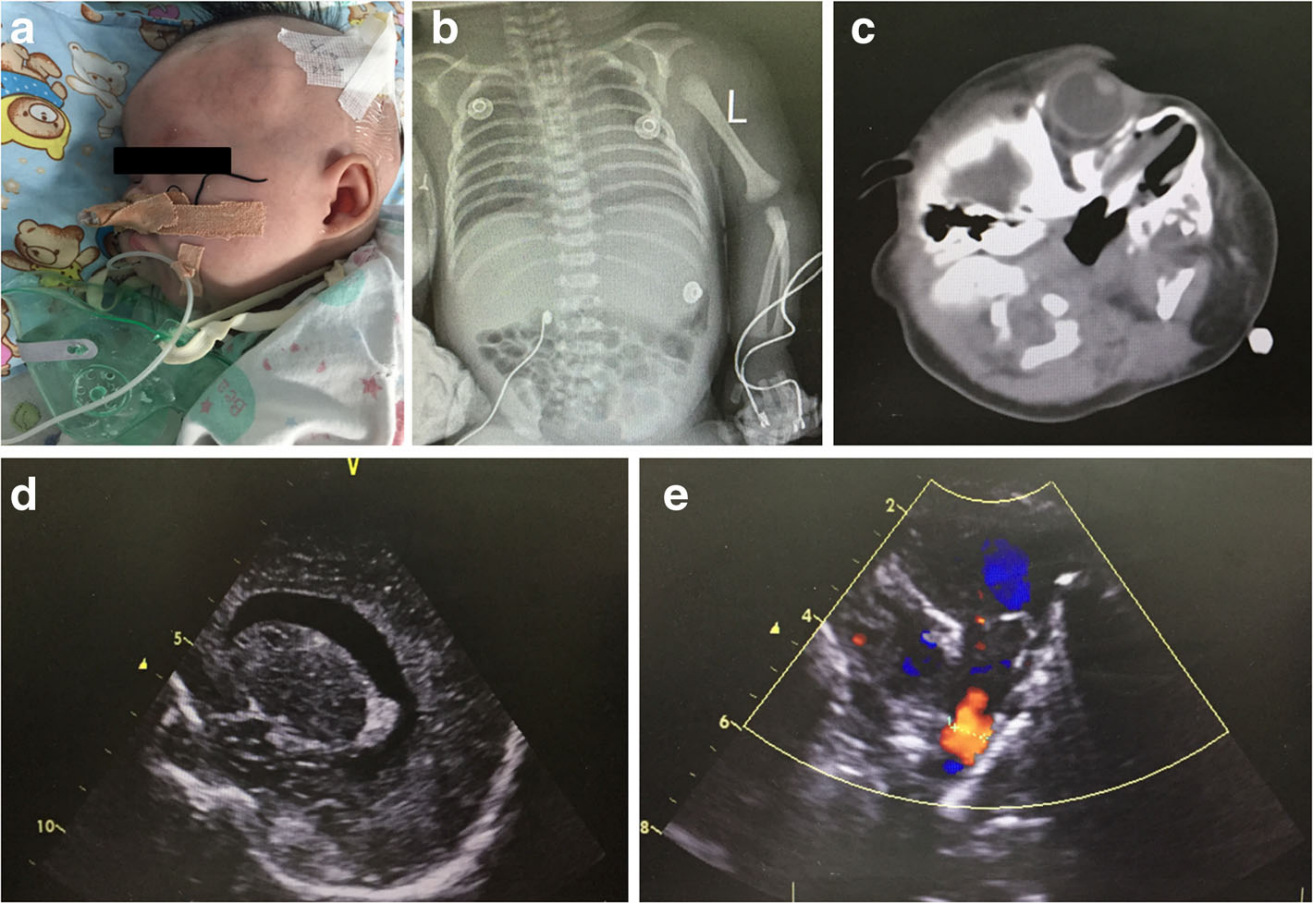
a Patient did posterior nostril plasty operation by nasal endoscope and put silicone tube in one month. b Chest radiograph at 3 days. c Computed tomography revealed bilateral choanal atresia and semi-circular canals as an insufficient inflatable structure. d Craniocerebral ultrasound showed bilateral lateral ventricle dilatation. e Echocardiography showed atrioventricular septal defect (6.8 mm + 2.2 mm) and patent ductus arteriosus (3.6 mm)
